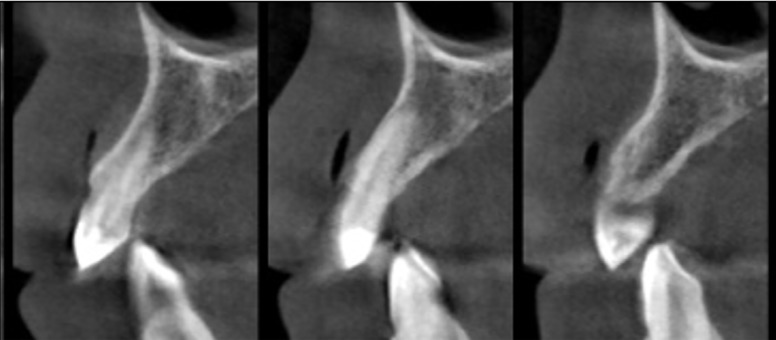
CBCT: Sagittal sections after a year: the surgical area shows signs of bone healing.
